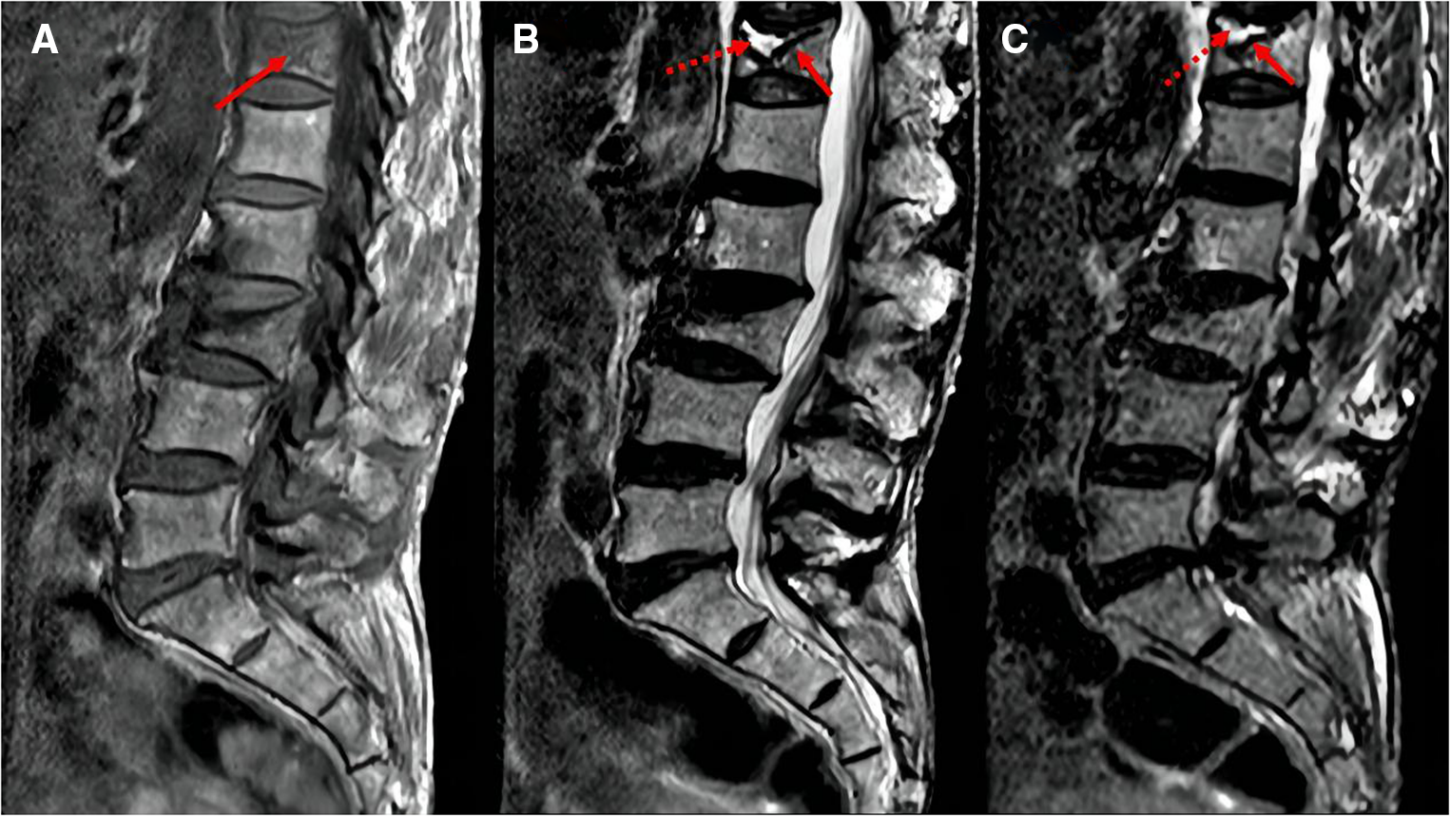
MRI images of intravertebral vacuum cleft. Hypointense on T1-weighted images (A, arrows); hyperintensity or hypointensity on T2-weighted images (B) or STIR images (C), depending on fluid (dotted arrows) or gas (solid arrows) filling.
